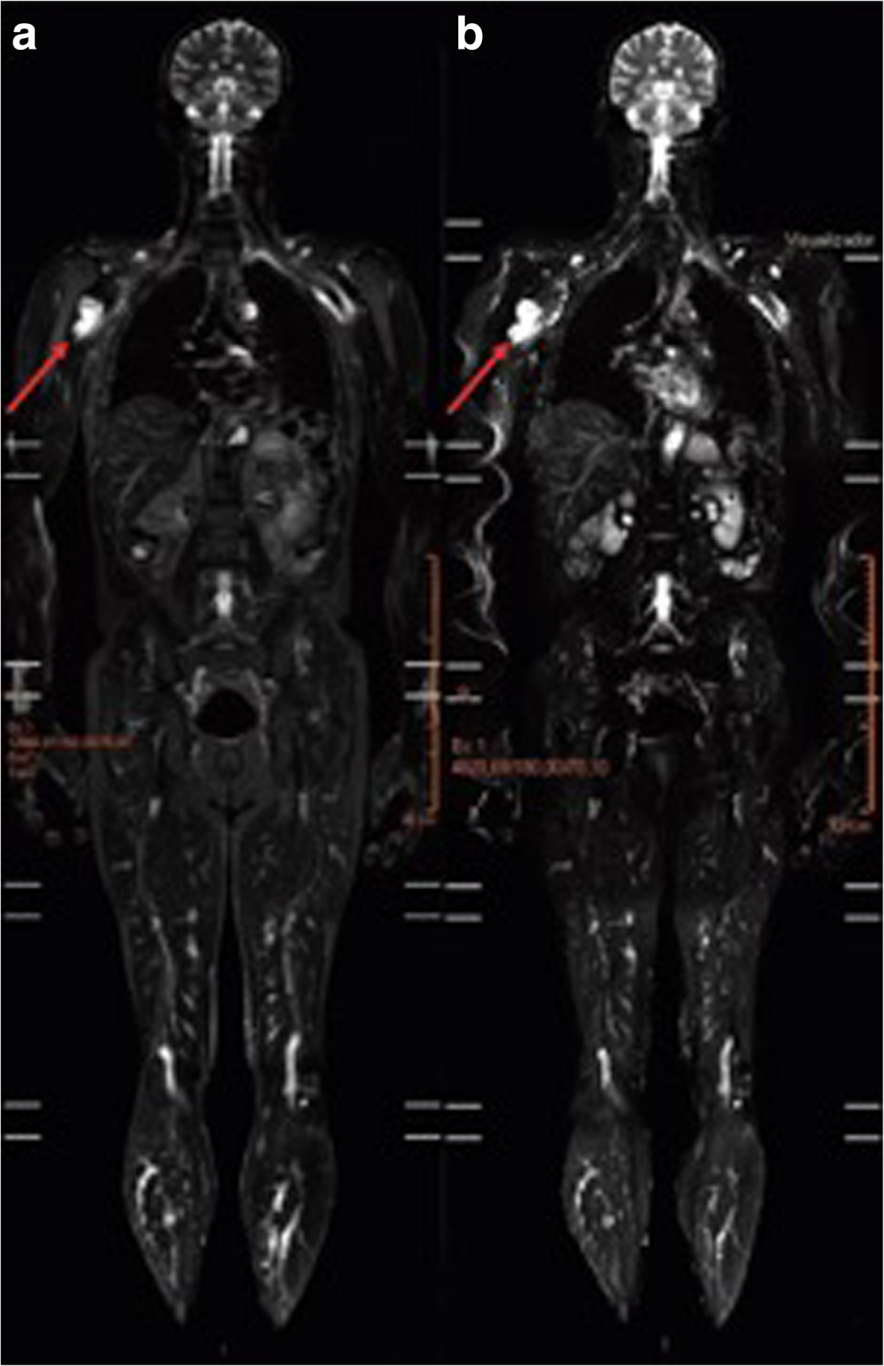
WB-MRI of LFS patient, Y0012T012. A STIR image (a) and diffusion-weighted imaging (b) from WB-MRI. An expansive lesion (48 × 34 mm^2^) in contact with the short head of the biceps was detected in the proximal segment of the right humerus. The lesion exhibited a hyperintense signal on STIR and restriction on diffusion-weighted imaging scans (indicated with arrows)
